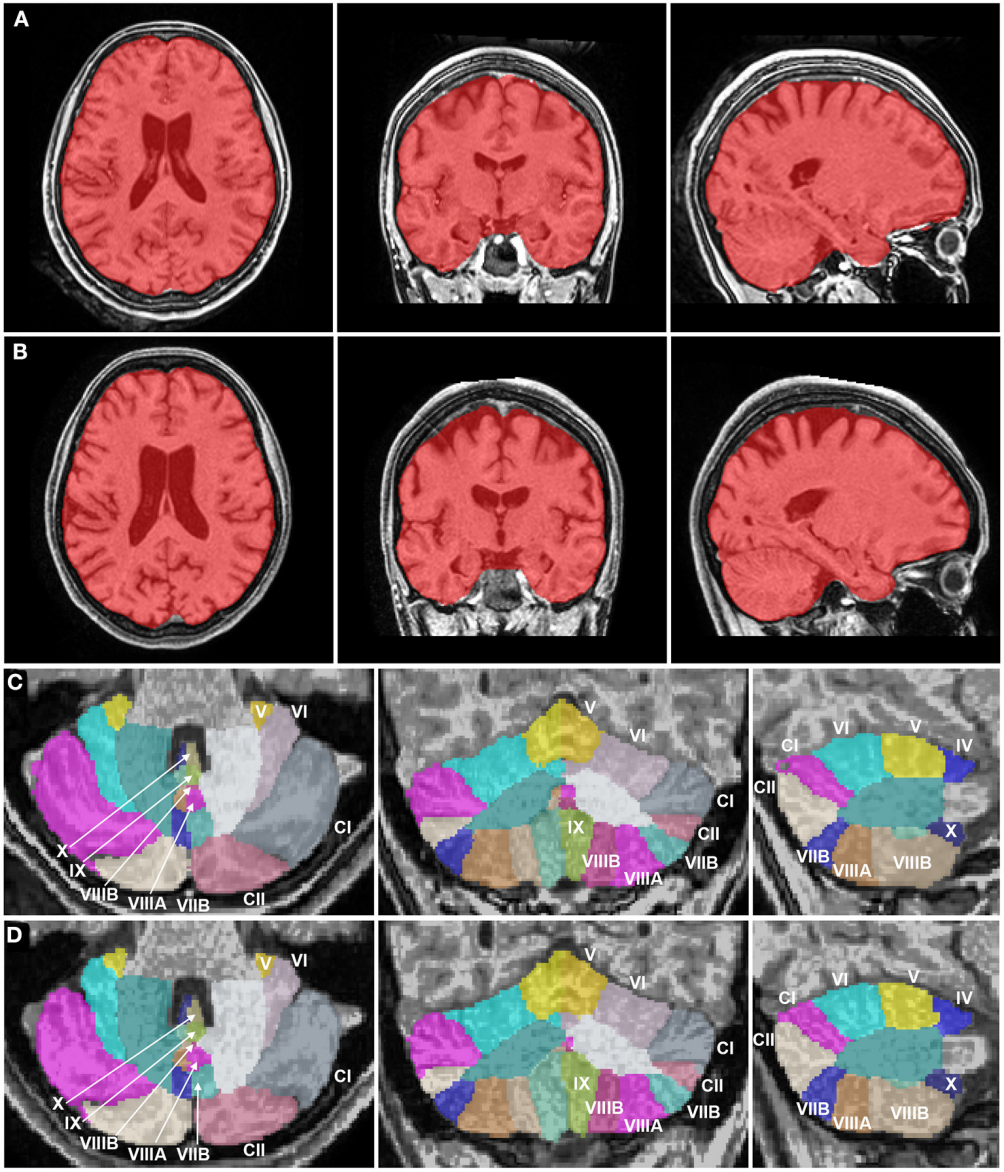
Brain magnetic resonance imaging segmentation maps of the patient with juvenile Huntington's disease at 18 years and 21 years. (A, B) Whole brain images (in red) of the patient in axial, coronal, and sagittal planes show an atrophy of the striatum at 18 years (A), progressing to a more diffuse process of atrophy, including subcortical structures and neocortical areas like the frontal and parietal lobes at 21 years (B). White matter volume was relatively preserved as compared to gray matter. (C, D) Cerebellum images of the patient in axial, coronal, and sagittal planes show a normal cerebellar volume and a volumetric stability at 18 years (C) and 21 years (D). The Roman numerals of the cerebellar lobules were added. CI, Crus I; CII: Crus II.
